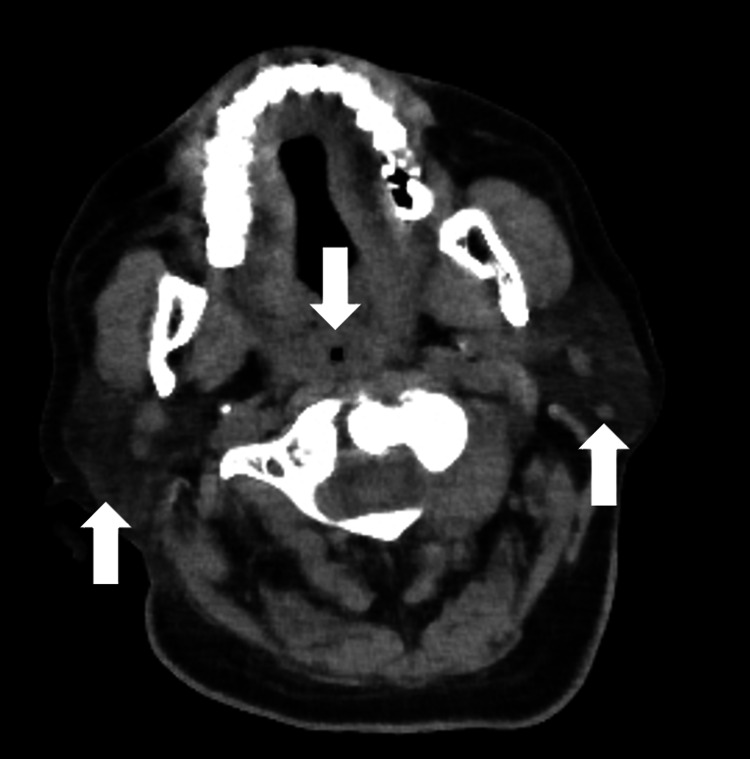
CT findings at the onset of local CRS, showing bilateral parotid gland swelling and airway stenosis. CT: computed tomography; CRS: cytokine release syndrome.
